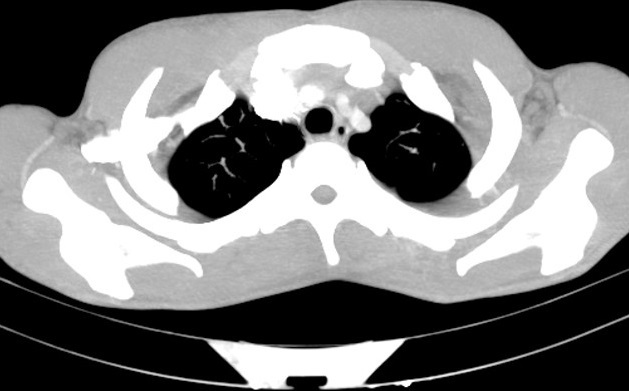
The patient’s CT pulmonary angiography
